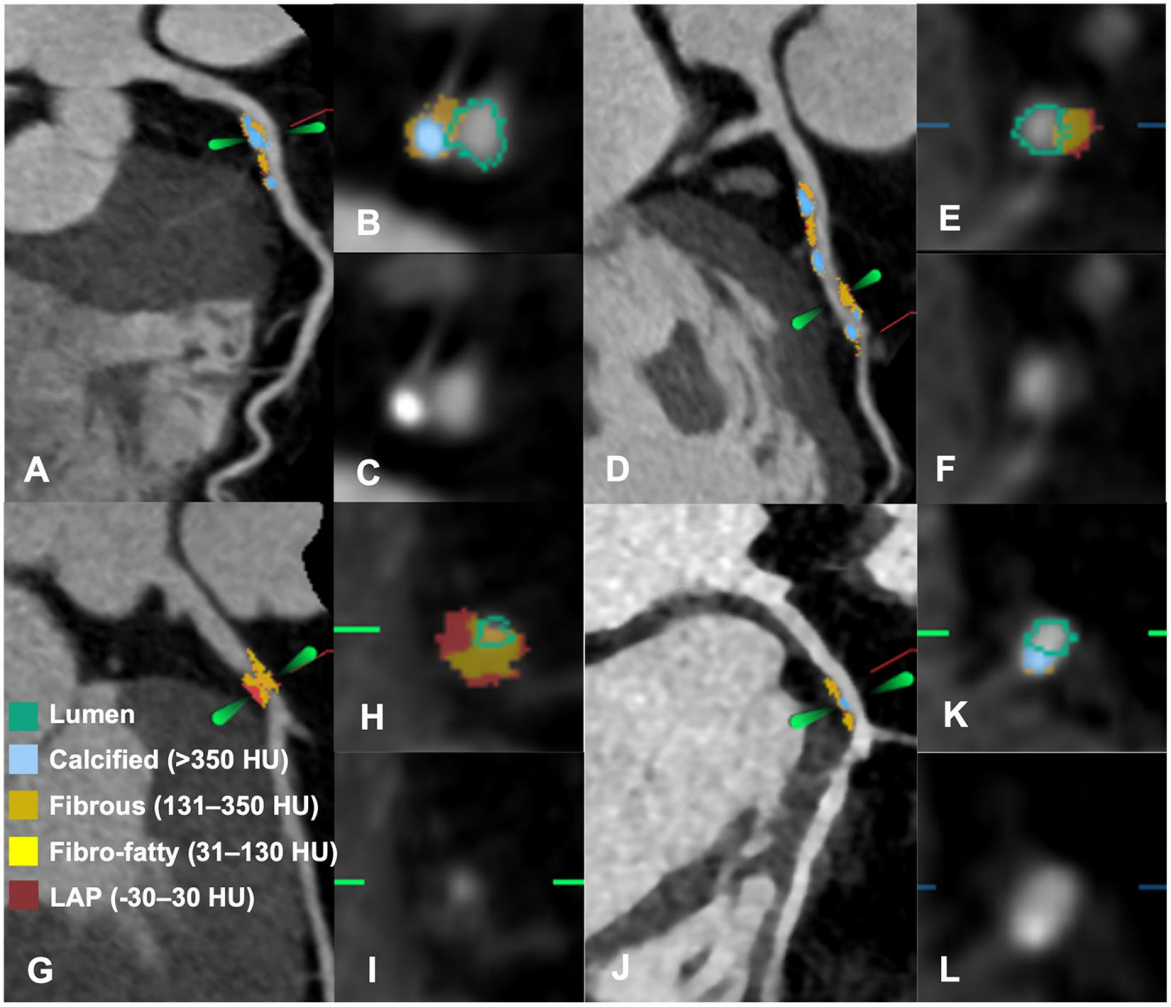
Representative cases of patients with and without plaque progression. (A–F) A 59-year-old woman with a baseline Lp (a) concentration of 112 mg/dL. (A) Baseline CCTA showed mild stenosis in the proximal LAD. (B,C) Cross-sectional reconstruction and component analysis of the target lesion. (D) Follow-up CCTA after 5.5 years revealed significant plaque progression, with an increase in LAP volume (7.9 mm^3^) and plaque volume (69.65 mm^3^). (E,F) Cross-sectional reconstruction and component analysis of the corresponding plaque. (G–L) A 55-year-old man with a baseline Lp(a) concentration of 17.4 mg/dL. (G) Baseline CCTA revealed severe stenosis in the proximal LAD. (H,I) Cross-sectional reconstruction and plaque component analysis of the target lesion. (J) At the 4-year follow-up, CCTA revealed regression of stenosis to a mild degree, with LAP volume and plaque volume decreasing by 20.16 mm^3^ and 85.33 mm^3^, respectively. (K,L) Cross-sectional reconstruction and component analysis at follow-up. CCTA, coronary computed tomography angiography; LAD, left anterior descending; LAP, low attenuation plaque; Lp (a), lipoprotein (a); PV, plaque volume.
